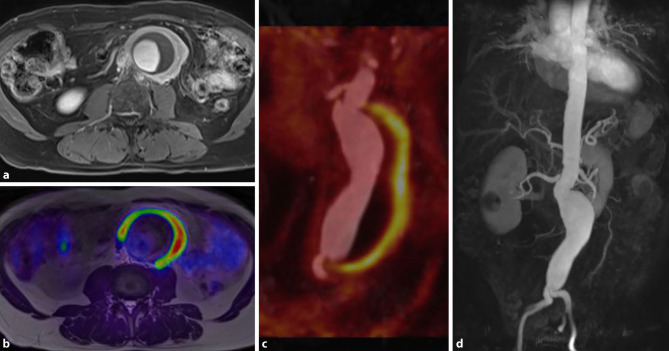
[^18^F]FDG PET/MR image of an inflammatory aortic aneurysm. Axial T1 vibe image after contrast shows the abdominal aortic aneurysm with vessel wall thickening and intraluminal thrombus formation (a). Fused images show pronounced [^18^F]FDG uptake within the thickened vessel wall as well (b, c). 3D rendering of the MR angiography shows the localized widening of the infrarenal aorta (d). The tracer activity of 289MBq corresponded to an effective dose of 7.8 mSv
